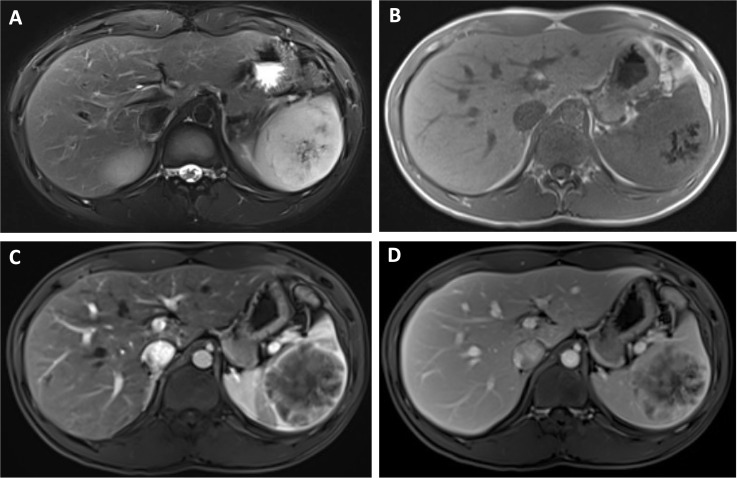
Axial MRI demonstrates that the mass appears slightly hyperintense on T2-weighted imaging (A) and slightly hypointense on T1-weighted imaging (B). On contrast-enhanced sequences, the lesion shows marked heterogeneous enhancement during the arterial phase (C), followed by progressive fill-in during the venous phase (D).
